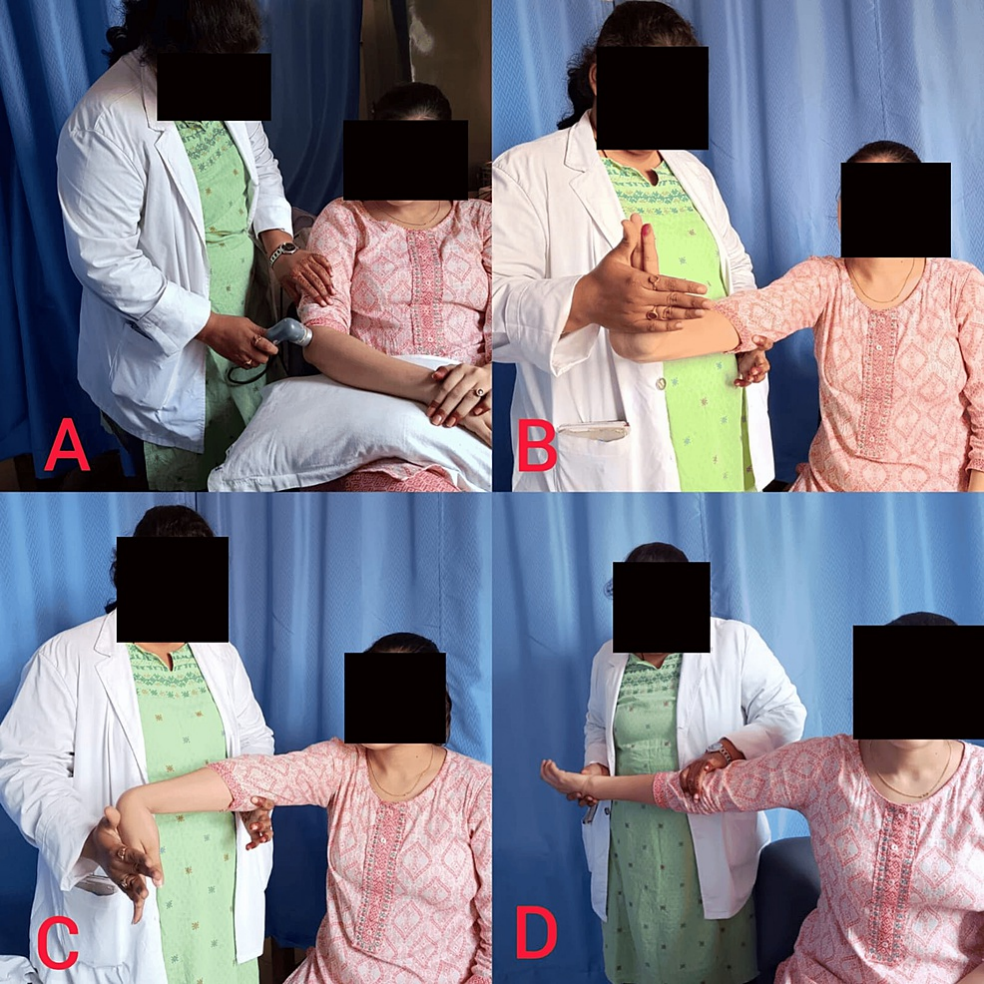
(A) Ultrasound. (B) Wrist extension stretching. (C) Wrist flexion stretching. (D) Cyriax technique
